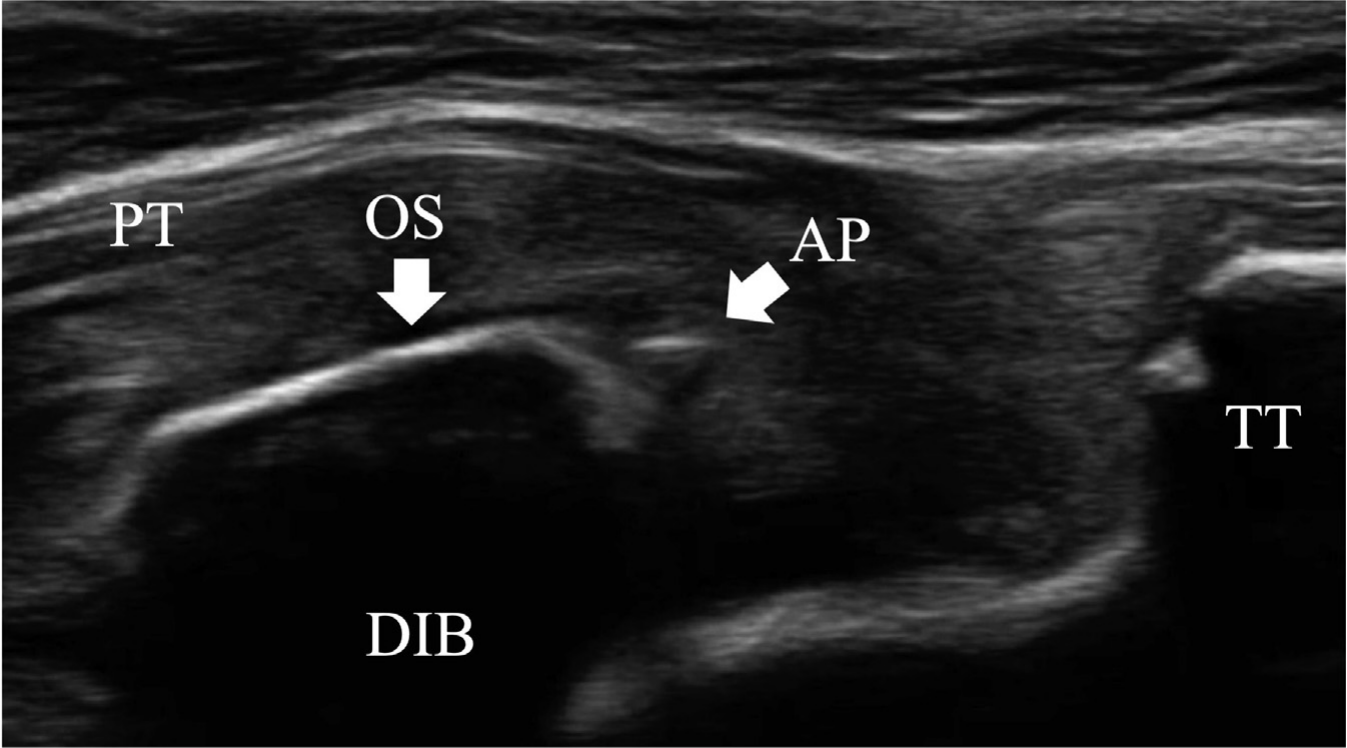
Ultrasound image while touching an ossicle with an arthroscopic probe. The ossicle is identified by touching it with an arthroscopic probe in the ultrasound image. (AP, arthroscopic device; DIB, deep infrapatellar bursa; OS, ossicle; PT, patellar tendon; TT, tibial tuberosity.)
